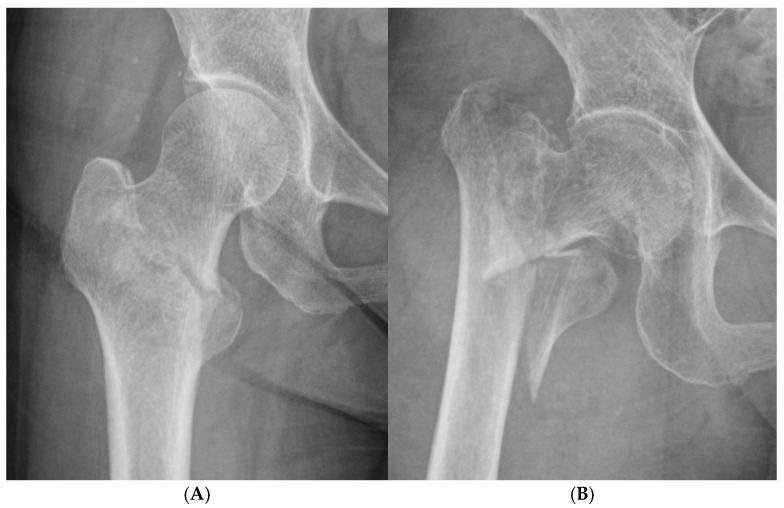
Radiographic classification of intertrochanteric fractures. (A) Simple two-part fracture with intact medial cortex. (B) Comminuted fracture with disruption of the posteromedial cortex.
